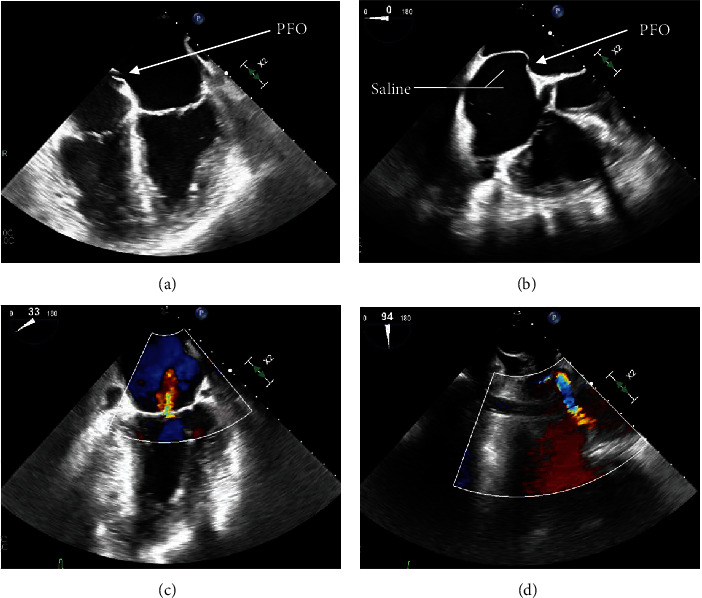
Transesophageal echocardiography revealing the patent foramen ovale (PFO). (a, b) The PFO (white arrow) in different views. (b) The leakage of saline contrast across the atria. (c, d) The shunting/mixing of blood through the PFO.
